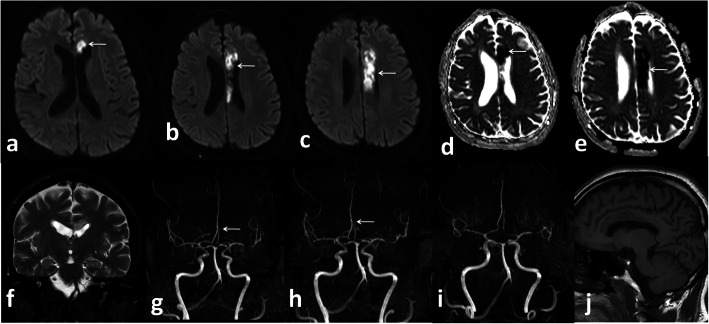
Female patient 54 years old. Left ACA territory infarction. DWI (white arrows in a,b,c), ADC map (white arrows in d and e), MRA defective A3 segment of left ACA (white arrows in g and h)
